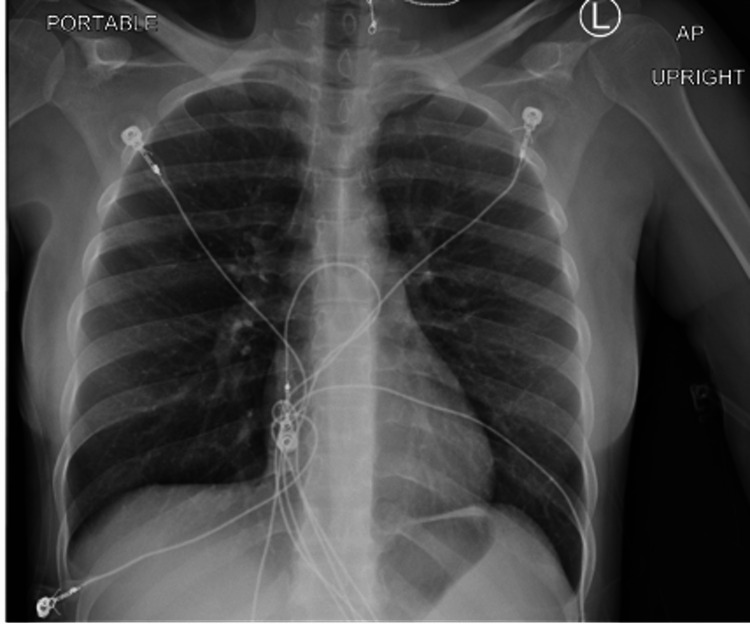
Chest x-ray on presentation without lung infiltrates
